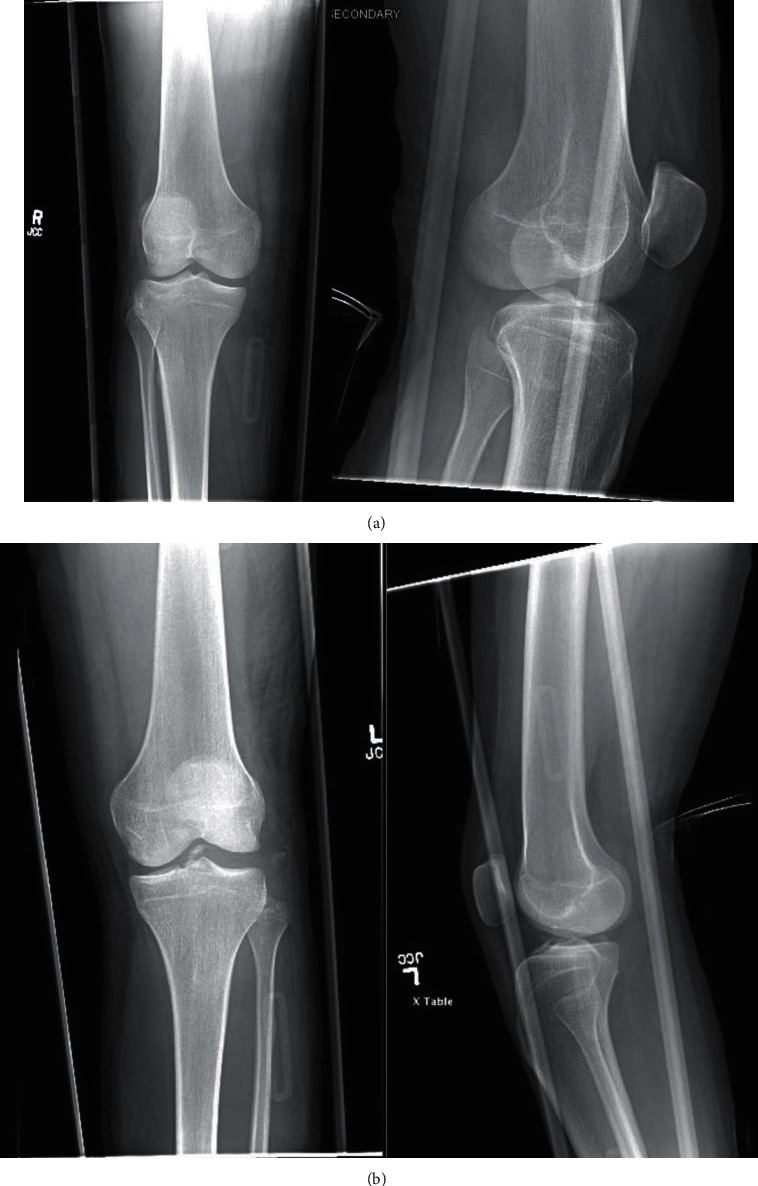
(a) Postreduction X-rays of the right knee. (b) Postreduction X-rays of the left knee.
